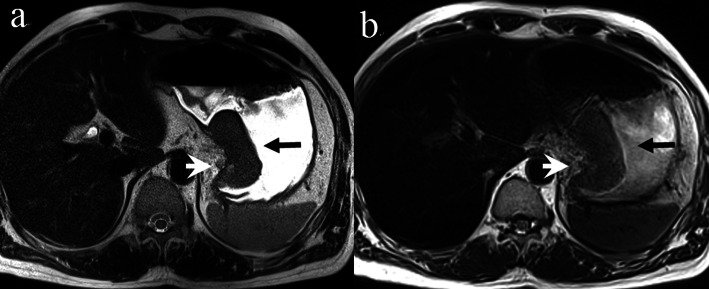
Comparison of two T2-weighted MR images of a patient with protrude moderately differentiated adenocarcinoma involving the lesser curvature of the stomach showing the superiority of HASTE (a) over BLADE (b). The intermediate-signal-intensity gastric mass can be seen on both images (arrow). Image artifacts were rated as“not hampering image evaluation” (score 2) for T2- HASTE, but rated as“hampering image evaluation” (score 1) for T2-BLADE. Lesion conspicuity was rated as“excellently delimitable” (score4), and “50–75% of borders definable” (score3), respectively. HASTE image showed the outer boundary remained smooth and a small, round lymph node next to the lesion (arrowhead), while due to the artifacts of BLADE image, there appeared to be an irregular/nodular outer border with perigastric fat infiltration (arrowhead), which may lead to incorrect staging
